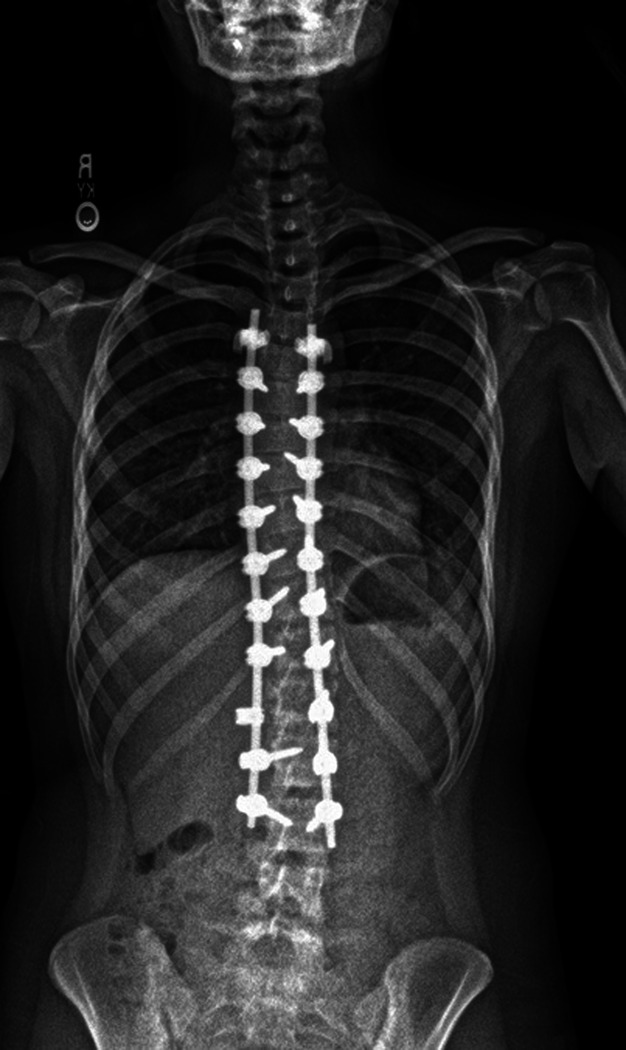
Postoperative radiograph of a patient who received erector spinae plane block with liposomal bupivacaine.
